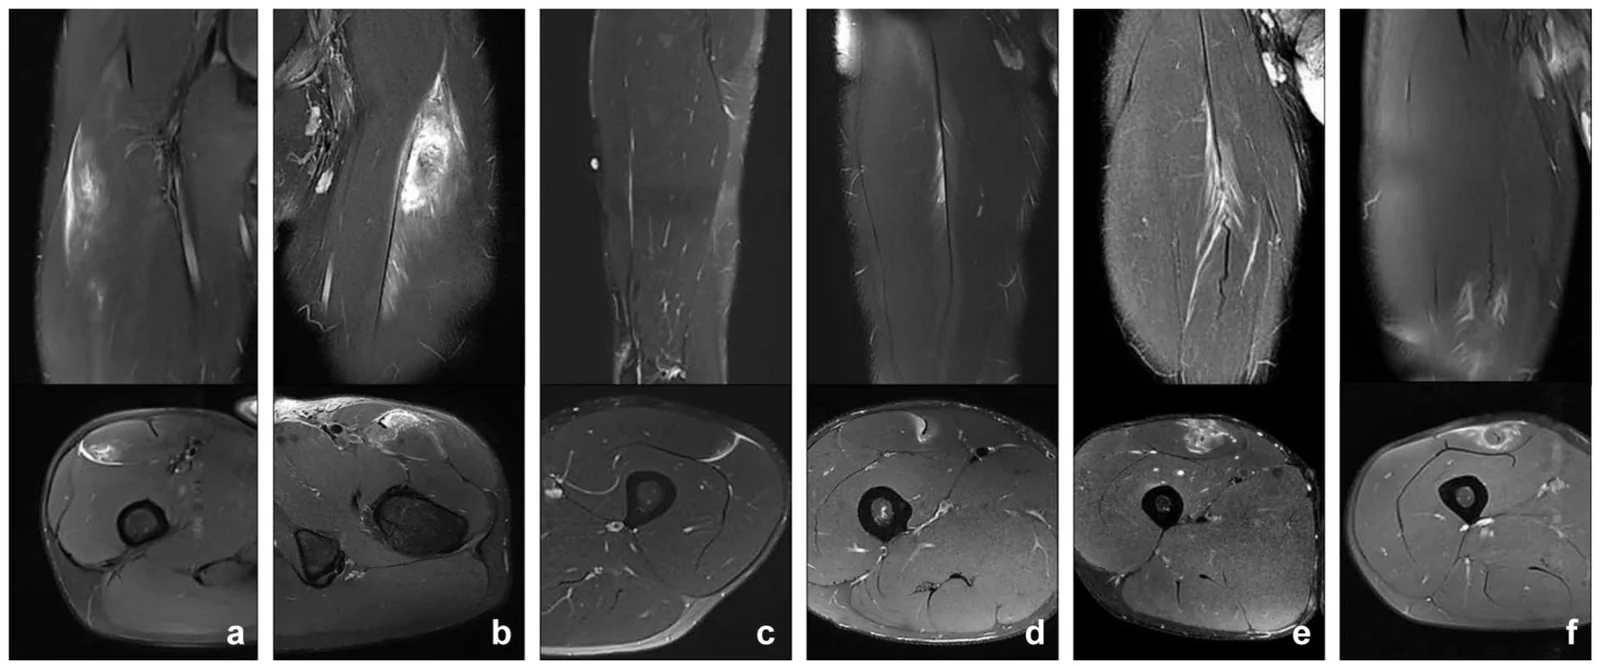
MRI of rectus femoris injuries located at various anatomical locations. (a) Posterolateral myofascial injury with intermuscular hematoma. (b) Anterior aponeurosis strain with mild AD and intramuscular hematoma. (c) Posterior lateral aponeurosis strain with mild AD. (d) Central aponeurosis myoaponeurotic strain with no AD. (e) Central aponeurosis strain with severe AD and waviness. (f) Primary degloving injury with retraction and associated waviness of central aponeurosis. AD, aponeurotic disruption; MRI, magnetic resonance imaging.
